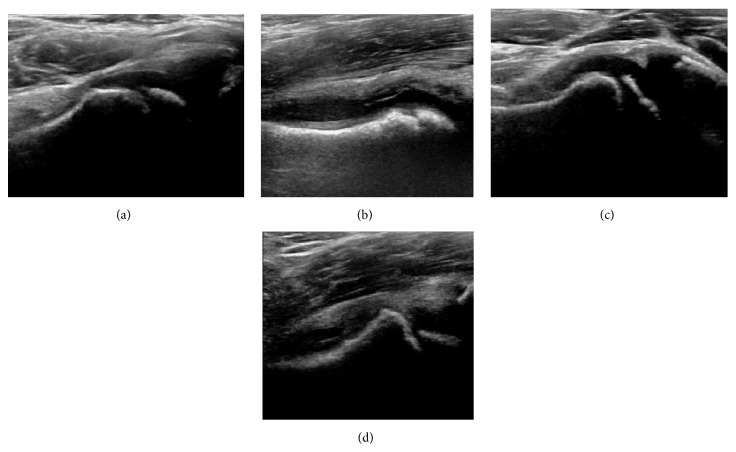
(a) Normal ultrasound appearance of the femoral head-neck junction. (b) Joint effusion in transient synovitis of the hip. (c) Flattening of the femoral head in a patient with Perthes disease. (d) Step in the femoral head-neck junction in a patient with SCFE.
